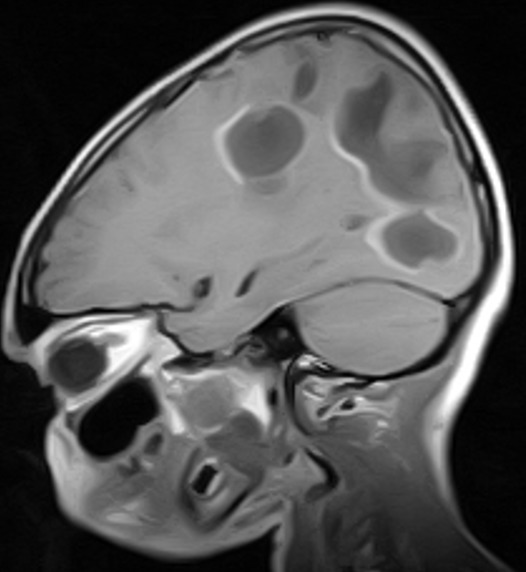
(MRI at presentation) is a post contrast T1 w parasagittal sequence showing multifocal, irregular hypointense areas with enhancing walls in the parieto-occipital region
